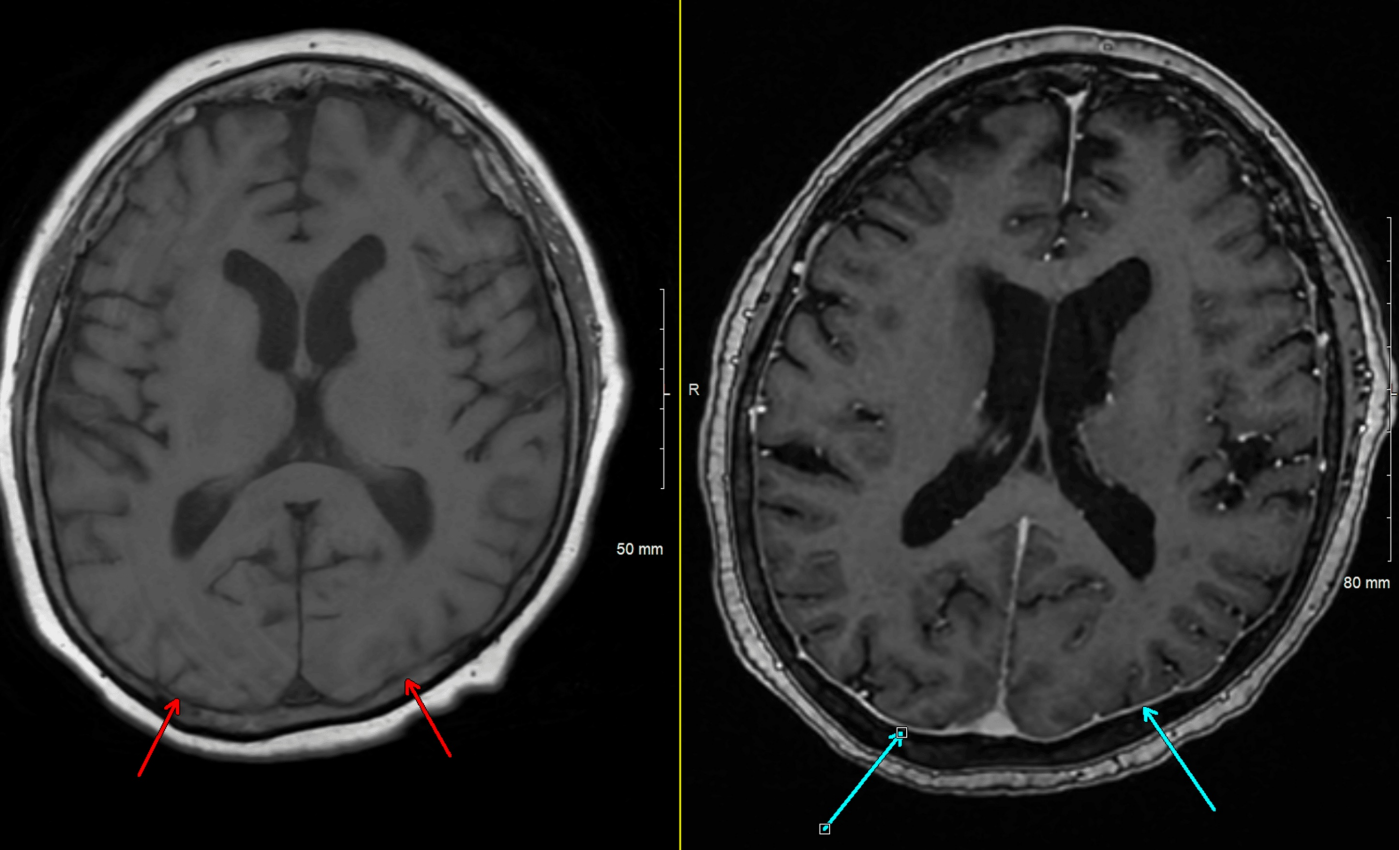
No abnormal enhancement Finding: Axial pre- (red arrow) and post-contrast T1 (blue arrow) MRI of the brain showing no abnormal enhancement in occipital lobes corresponding to the FLAIR hyperintensity. Technique: 1.5 T MRI, pre- and post-contrast T1 sequence. FLAIR: fluid-attenuated inversion recovery; MRI: magnetic resonance imaging
